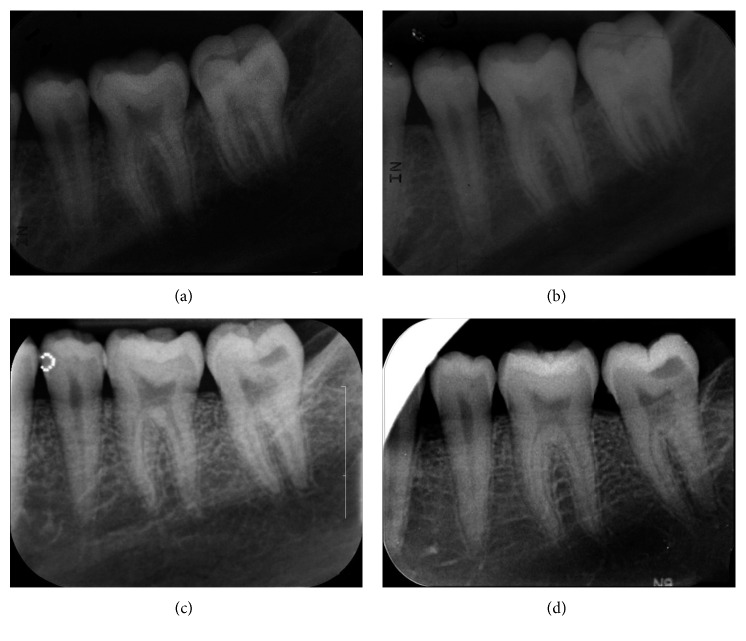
Periapical radiographs of the PEIR-affected tooth (permanent mandibular left second molar) when the patient was (a) 10 years, 11 months old, (b) 11 years, 5 months old, (c) 15 years, 3 months old, and (d) 17 years, 4 months old. The tooth is in the normal path of eruption, has normal crown formation, and has had continuing root formation since the first radiograph.
